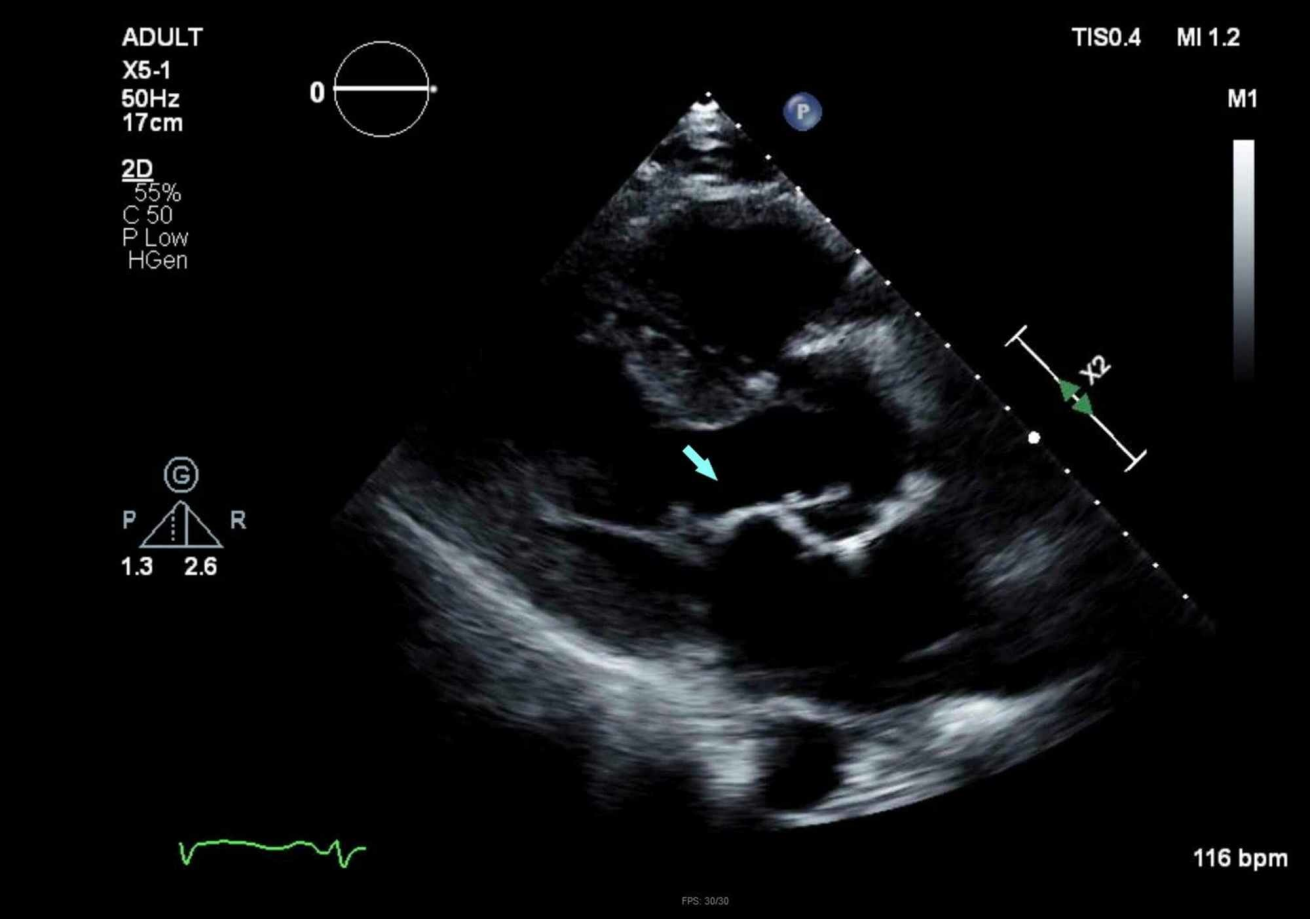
Transthoracic echocardiogram (TTE) revealing the mitral valve (arrow)
